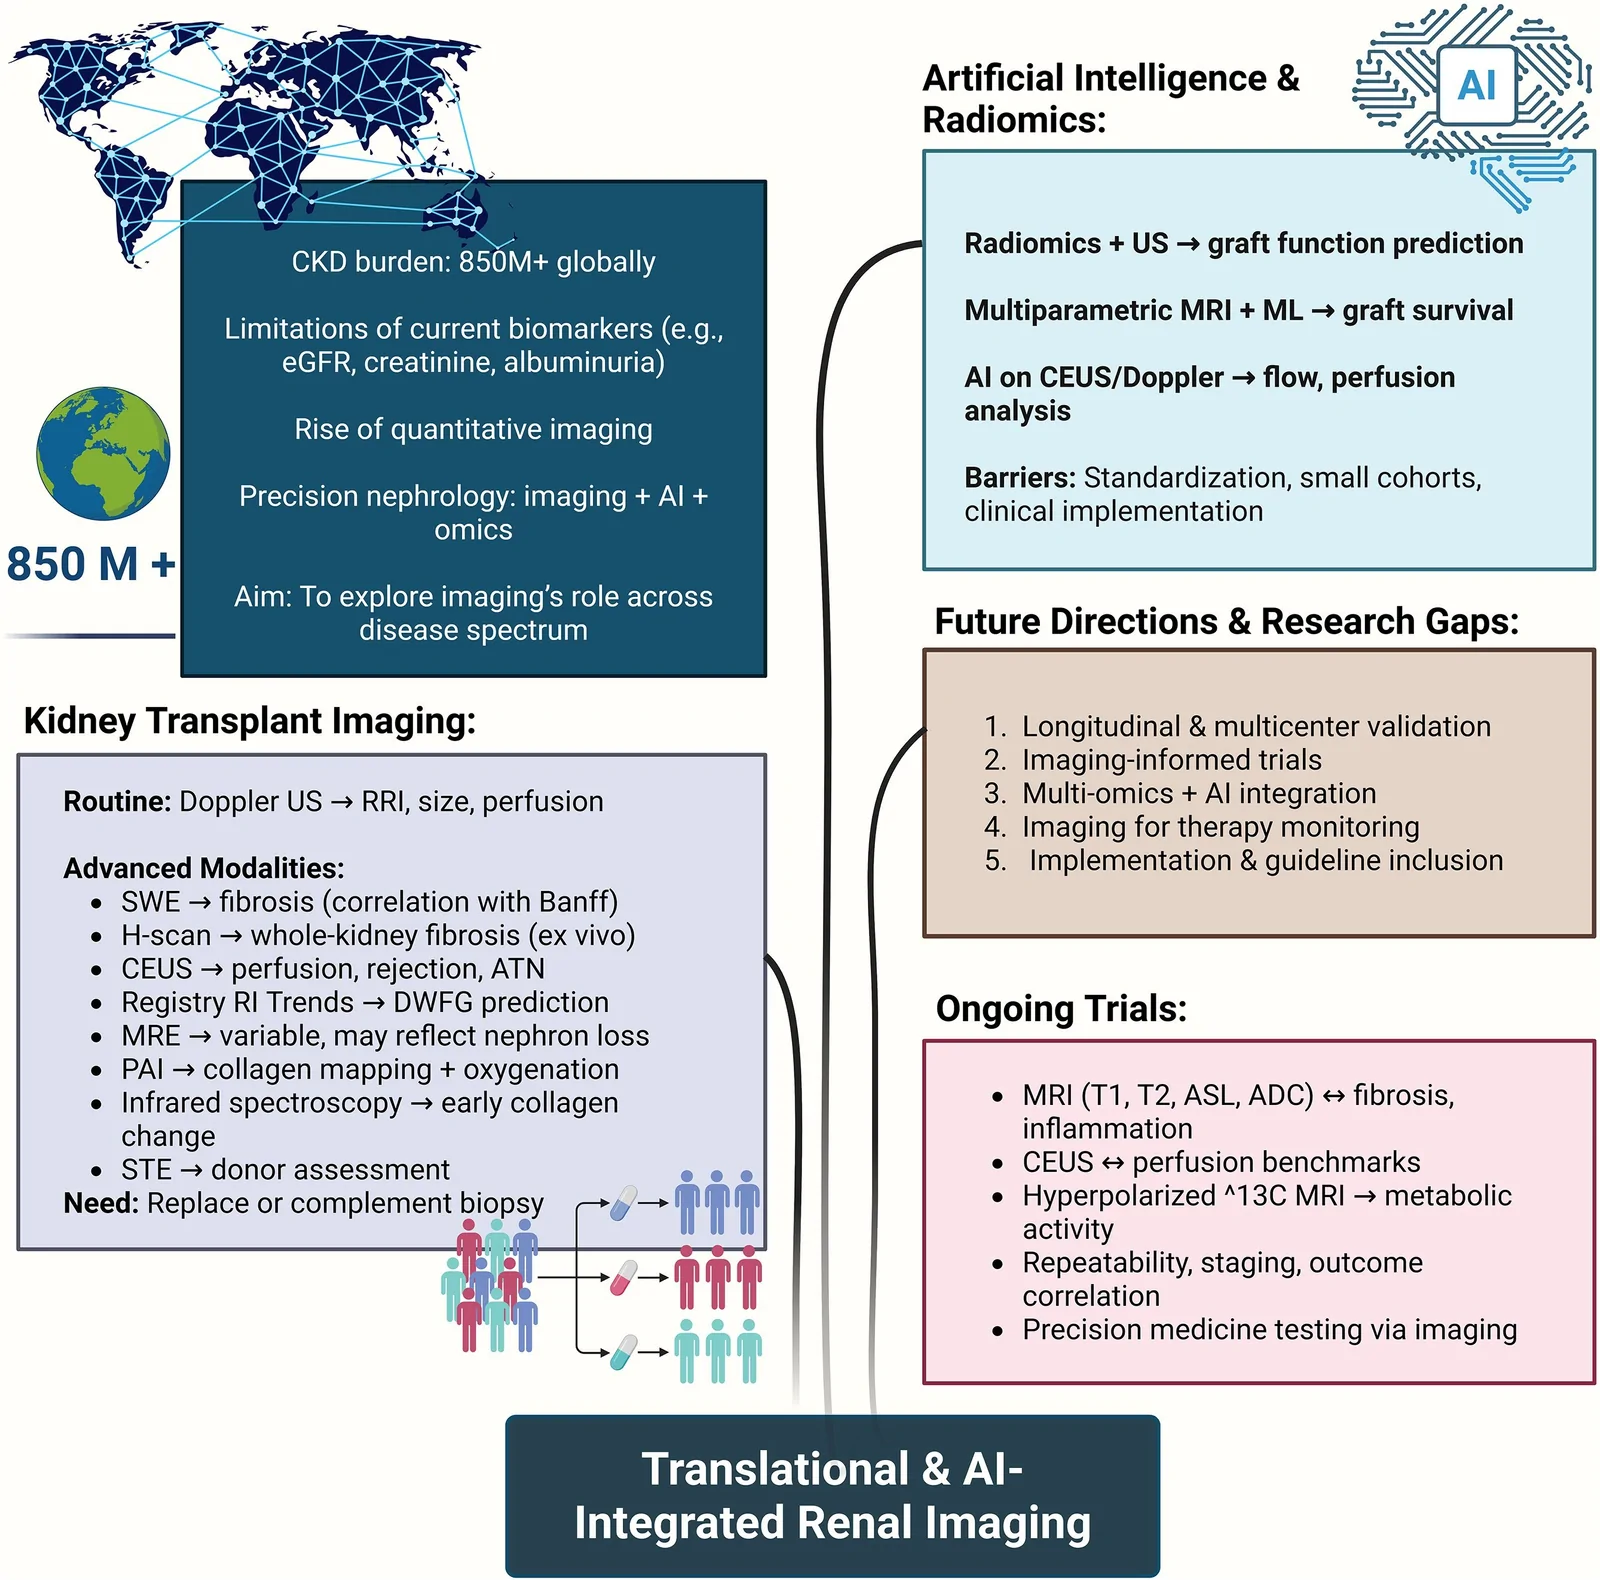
Quantitative and functional renal imaging across the CKD spectrum. Quantitative MRI and ultrasound modalities enable early detection of key pathophysiological changes—fibrosis, hypoxia, and microvascular rarefaction—before traditional biomarkers (eGFR, albuminuria) decline. Techniques, such as, BOLD MRI, ASL, IVIM-DWI, T1 mapping, and elastography characterize oxygenation, perfusion, and tissue stiffness across chronic, diabetic, and metabolic kidney diseases. Early imaging-based detection and risk stratification may facilitate timely therapeutic action, slowing or halting CKD progression. Abbreviations: ADC, apparent diffusion coefficient; AI, artificial intelligence; ARFI, acoustic radiation force impulse; ASL, arterial spin labeling; BOLD, blood oxygenation level-dependent; CEUS, contrast-enhanced ultrasound; CKD, chronic kidney disease; CVD, cardiovascular disease; DKD, diabetic kidney disease; DWFG, death with functioning graft; eGFR, estimated glomerular filtration rate; IVIM-DWI , intravoxel incoherent motion diffusion-weighted imaging; MRA. magnetic resonance angiography; MRE. magnetic resonance elastography; MRI. magnetic resonance imaging; MRS. magnetic resonance spectroscopy; PAI. photoacoustic imaging; PDFF. proton-density fat fraction; PRS. polygenic risk score; *R*_2_*. transverse relaxation rate; RI. resistive index; RRI, renal resistive index; RSF, renal sinus fat; SMI, superb microvascular imaging; SWE, shear-wave elastography; STE, strain-tensor elastography; T1/T2, relaxation times; and US, ultrasound.
